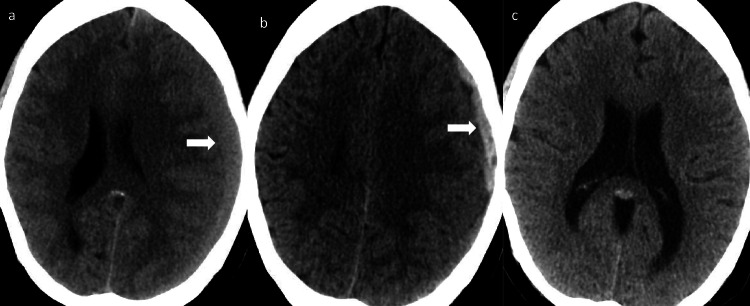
a: CT head on admission showed 14 mm left frontal-parietal subacute subdural hemorrhage with 9 mm left to right midline shift. b: One-month post middle meningeal artery embolization CT head showed 10 mm acute left frontal subdural hemorrhage. c: Six months post deep temporal artery embolization CT head showed completely resolved subdural hemorrhage.
